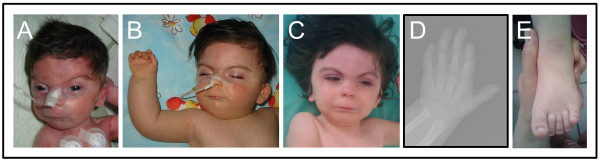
Phenotype of the patient. ( A) Frontal view of the patient in the first week of life. ( B) Frontal view and right hand of the patient at the age of 2 years and 10 months; and ( C) at 3 years. ( D) Roentgenogram of the left hand at the age of 3 years and 6 months and ( E) left foot of the patient at the age of 3 years. Note typical CdLS facial features seen in our patient included synophrys, arched eyebrows, long eyelashes, depressed nasal bridge and anteverted nares, long and flat philtrum, thin upper lip, downslanting corners of the mouth, low set and posteriorly rotated ears and microbrachycephaly ( A- C). Several mild musculoskeletal anomalies were noted including small hands and feet ( D and E), clinodactyly of the fifth finger and proximally placed thumb ( D).
